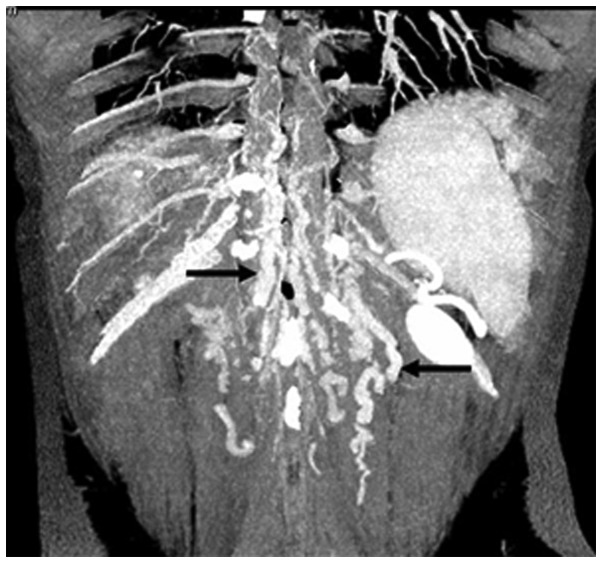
Superficial epigastric vein collaterals. Computed tomography angiography shows the expanded superficial epigastric vein collaterals (arrows).
